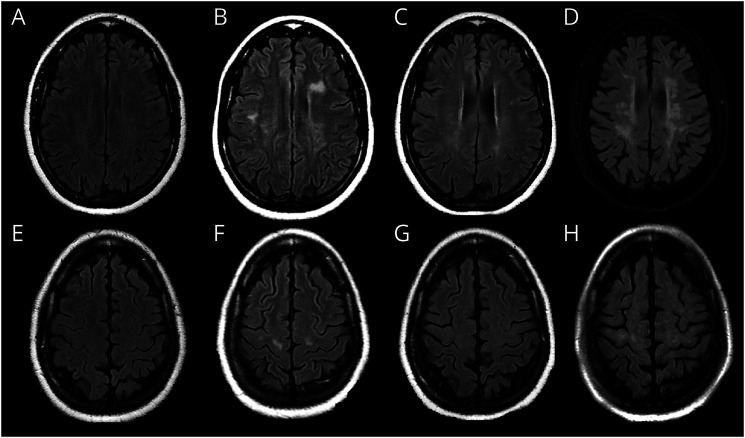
MR Imaging in a Patient With HTLV-1–Related Encephalopathy Serial axial FLAIR images at different time points over a 9-year period in a female patient with HAM and recurrent encephalopathic episodes. The first column (A and E) 9 years ago when the patient had established HAM demonstrates minimal subtle abnormal signal in the centrum semiovale. MRI 1 year later (B and F) shortly after an acute encephalopathic episode characterized by worsening upper limb weakness, diplopia, and ataxia demonstrates new bilateral lesions in the precentral white matter with additional deep and periventricular lesions in the frontal white matter. MRI 4 years later (C and G) demonstrates regression of the lesions with only subtle, Ill-defined residual abnormal signal. MRI 5 years later (D and H) during an episode of rapidly worsening HAM and impaired cognition demonstrates recurrence of the precentral lesions and new frontal white matter lesions.
